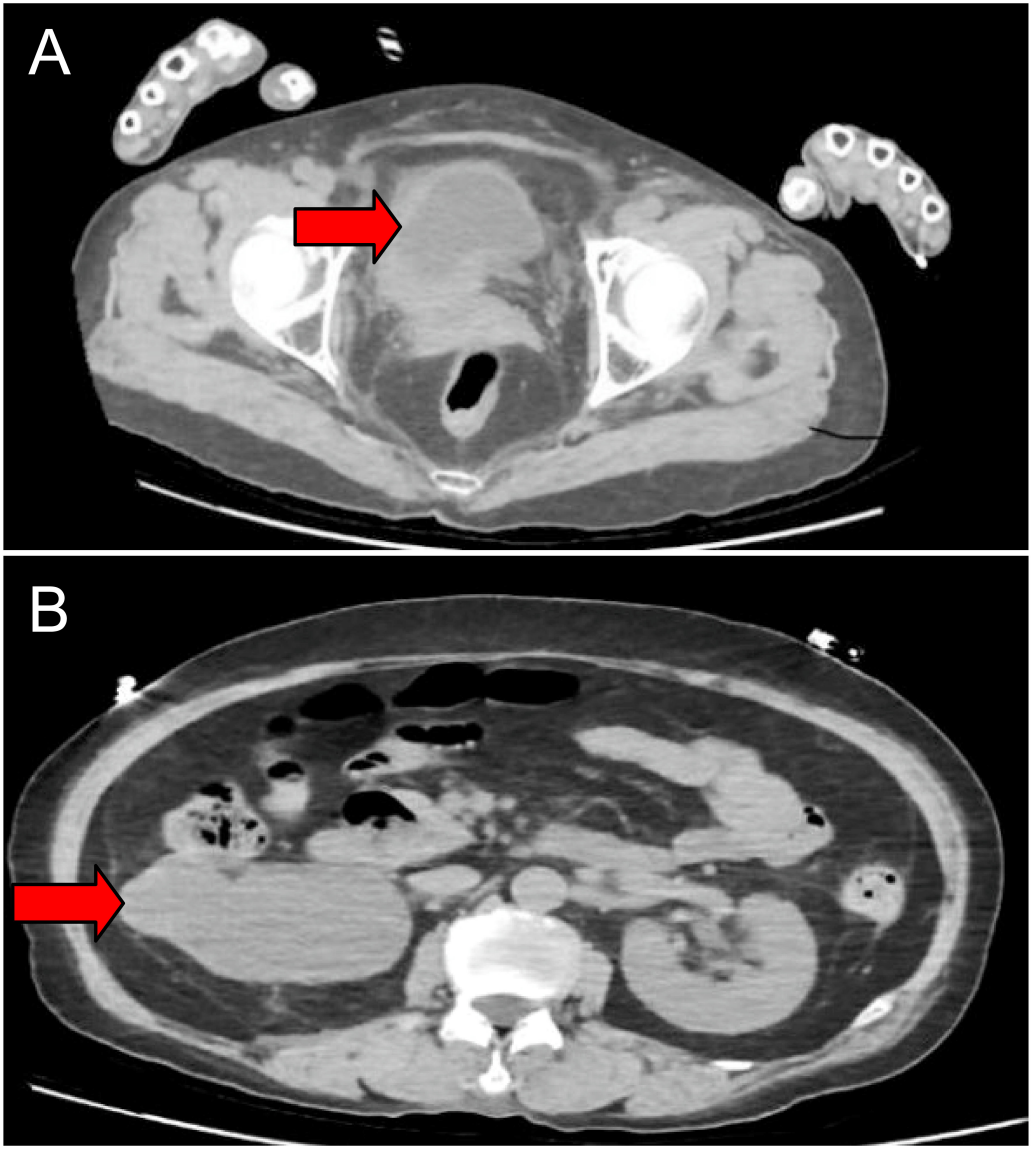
Imaging studies before treatment. CT showing thickening in the right lateral wall of the urinary bladder (cross-sectional) (A). Severe right hydronephrosis(cross-sectional) (B).
